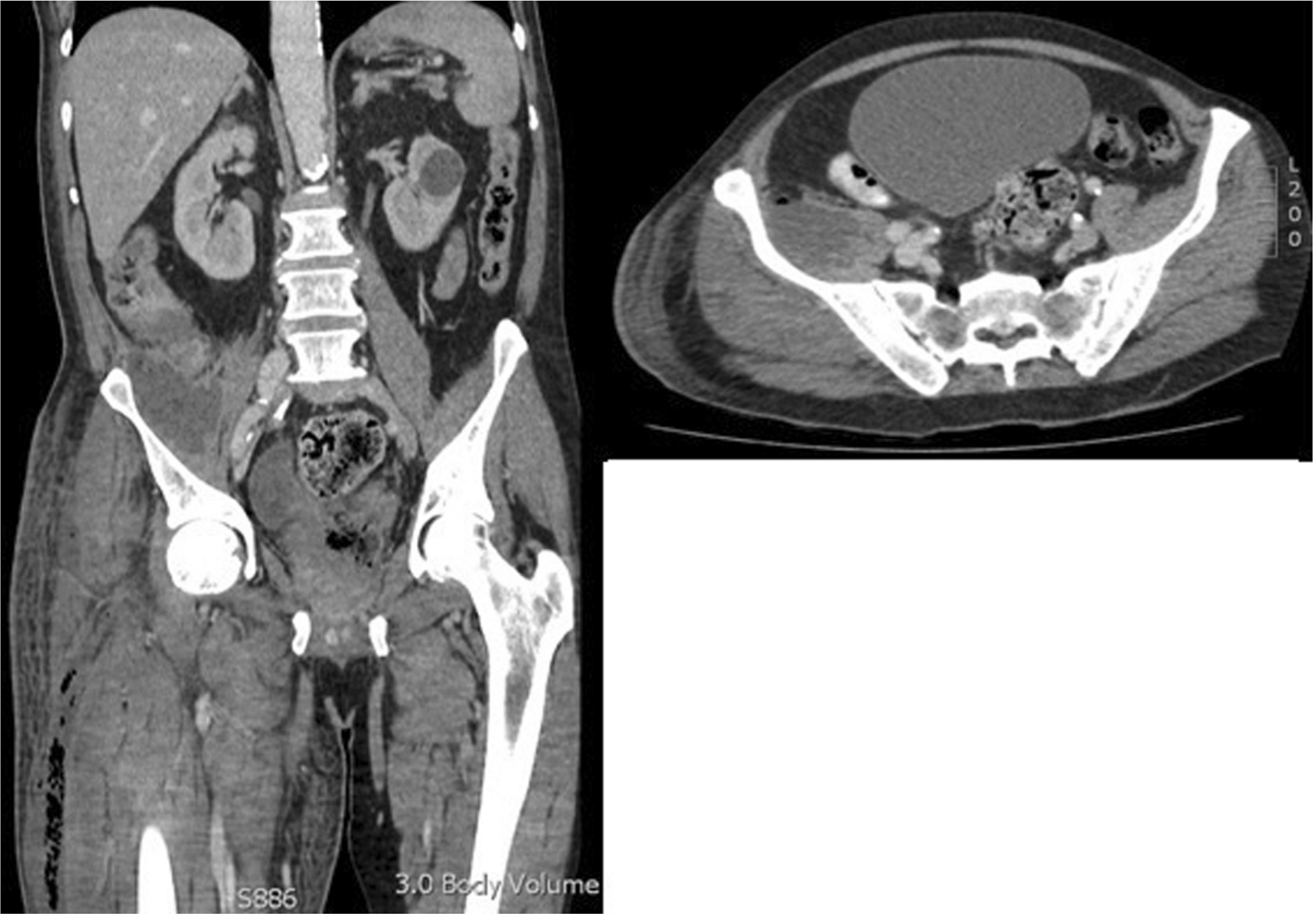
Computed tomography scan showing the perforated caecal carcinoma and free air tracking down the iliopsoas into the lower limb.
